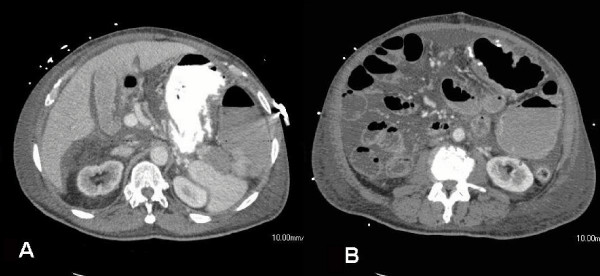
Post operative day six abdominal CT demonstrating pancreatic tail/splenic flexure mass and dilated colon proximal to splenic flexure.
